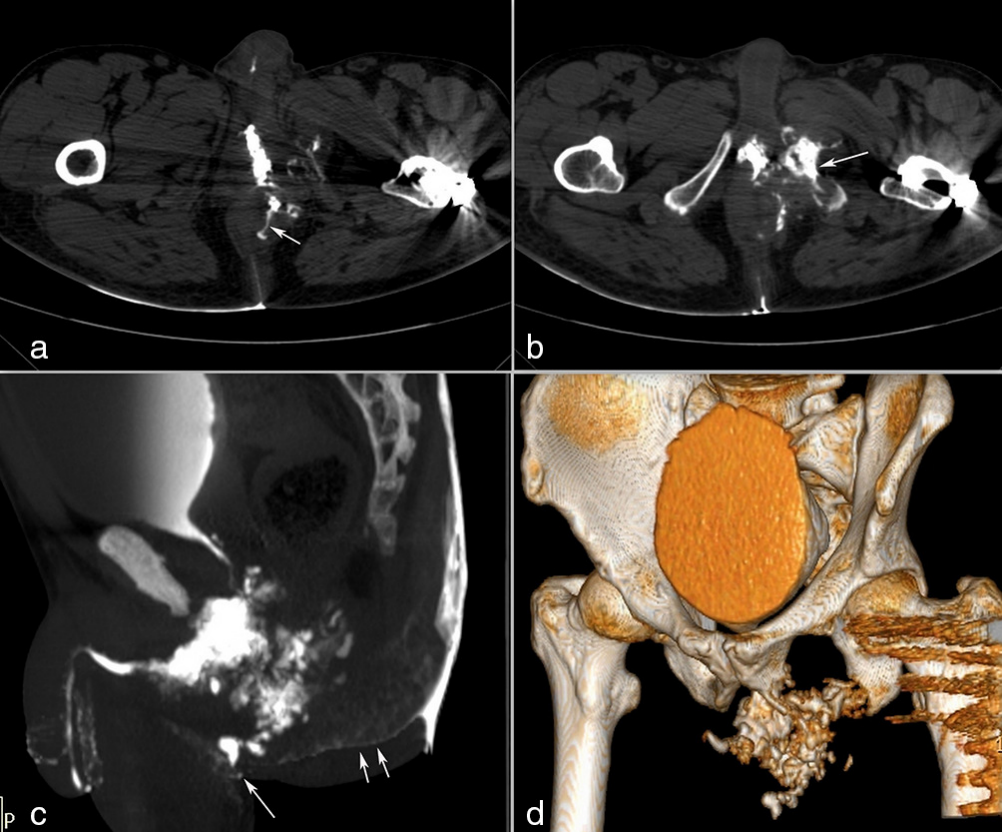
Post-operative axial retrograde CT urethrogram images (a and b) show extension of the injected contrast to intersphincteric planes of the anal canal (arrow in a) and a lytic lesion in the left inferior pubic ramus (arrow in b). The sagittal maximum intensity projection (c) and the volume-rendered (d) CT images show a complex periurethral collection communicating with the perineal skin (arrow in c) and spilling into the natal cleft (double arrow in c).
